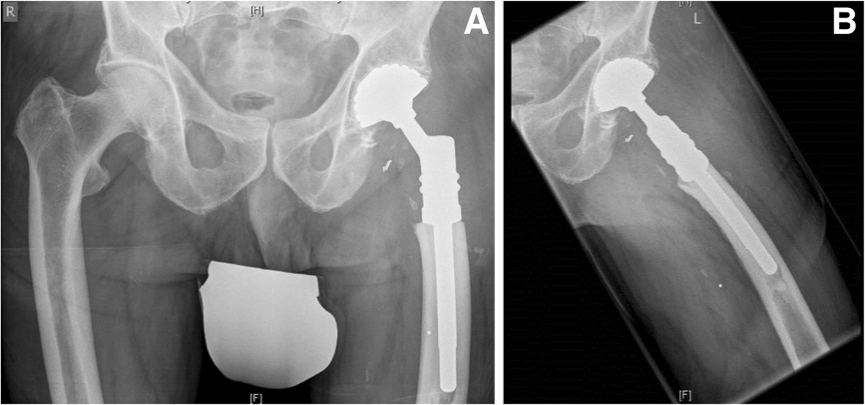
(A) and (B): Radiographs showing the typical post-operative findings after reconstruction with a modular tumor endoprosthesis: (A) anterior-posterior and (B) axial radiograph of the proximal femur of a 76-year-old male patient (see Figure
